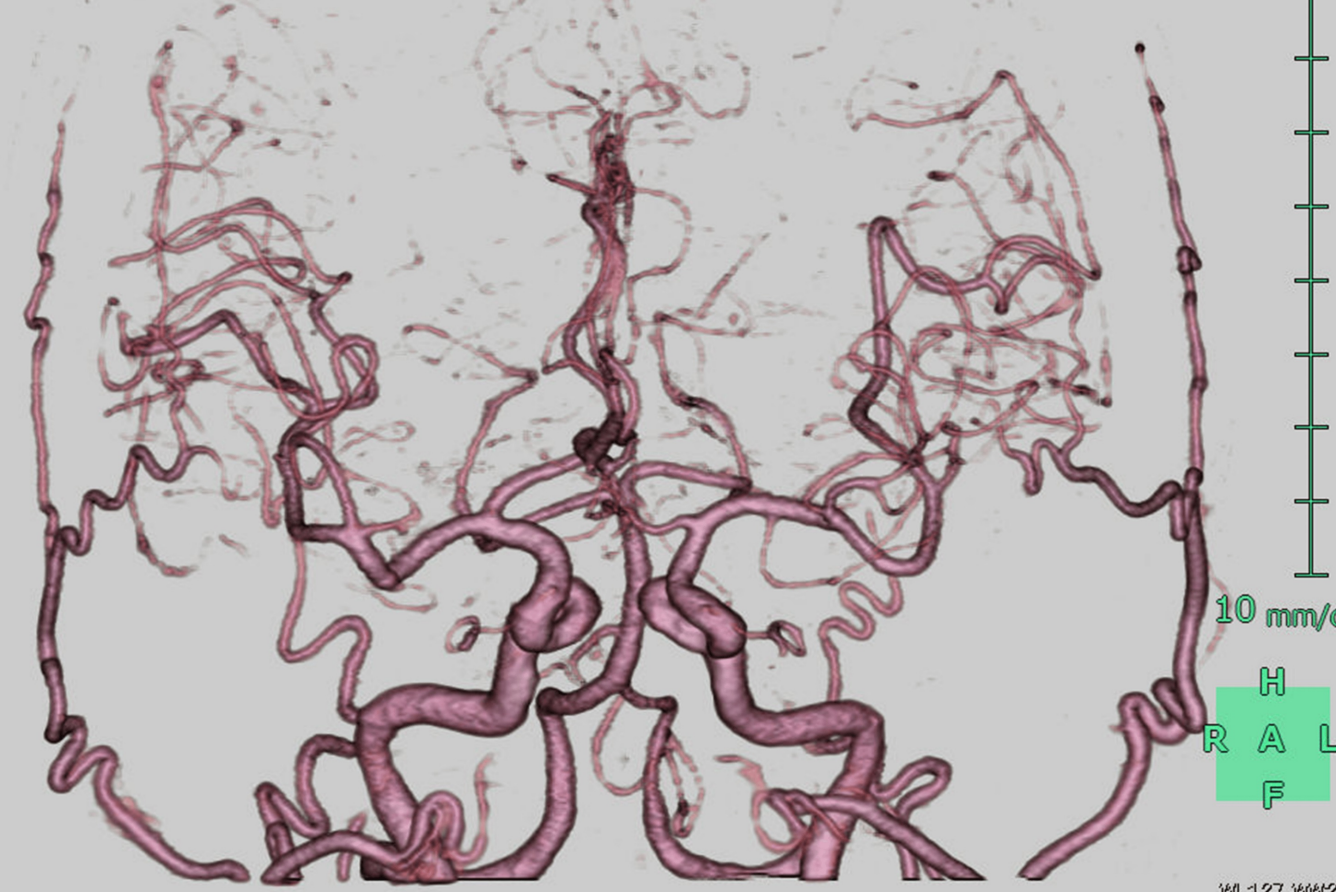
Three-dimensional computed tomography angiography (3D-CTA) (Case 1). No cerebral aneurysms or other vascular abnormalities were observed. Note: The blue color indicates orientation markers (anterior–posterior and right–left directions) and does not represent any pathological findings.
